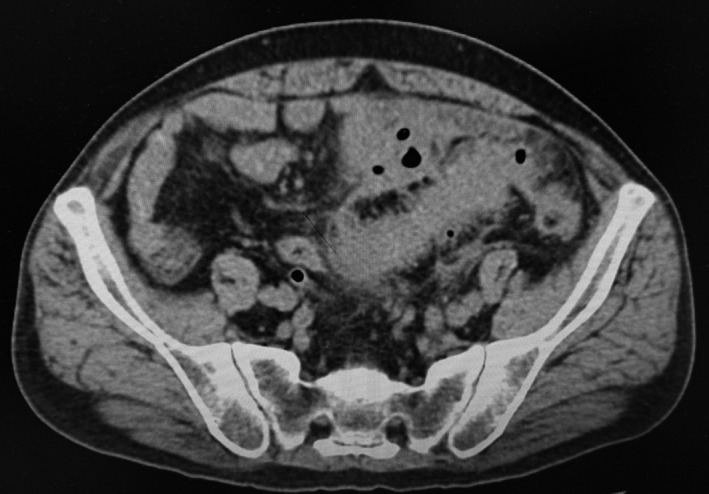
Computed tomography image shows pericolic abscess walled off by colon, mesocolon, bladder and pelvic peritoneum. This is Hinchey stage II. This patient was treated with antibiotics but failed. Sigmoid colon resection was carried out
